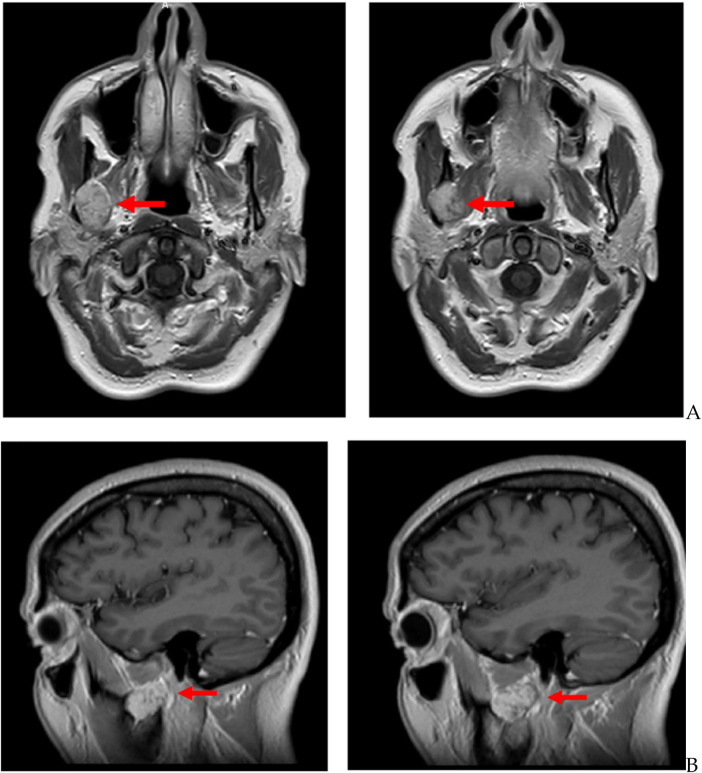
(A) preoperative MRI with a contrast medium; axial T1 fat sat sequence reveals an ovoid, lobed, encapsulated, and well-defined lesion measuring 2.5 cm in the right medial compartment of the masticator space, with heterogeneous T1 signal and late heterogeneous enhancement. There are no signs of invasion of the adjacent structures of anatomical spaces. (B) Preoperative MRI with a contrast medium; sagittal T1 fat sat sequence reveals an ovoid, lobed, encapsulated, and well-defined lesion measuring 2.5 cm in the right medial compartment of the masticator space, with a heterogeneous T1 signal and late heterogeneous enhancement. There are no signs of invasion of adjacent structures or anatomical spaces.
